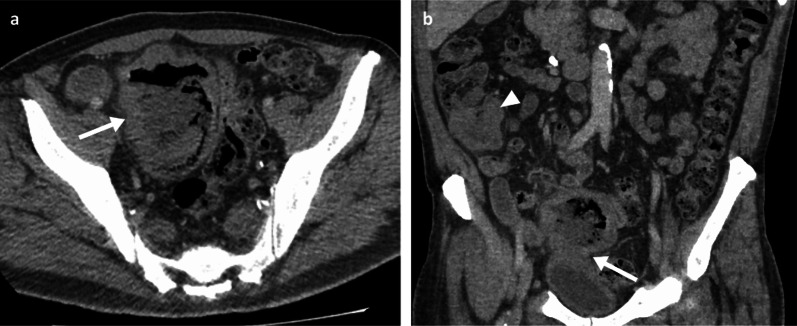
Portal phase contrast-enhanced CT axial and coronal (a, b) of a 71-year-old male patient demonstrating a large cavitating small bowel melanoma metastasis with urinary bladder tethering and dome invasion (arrows). Further separate caecal pole metastasis (arrowhead)
